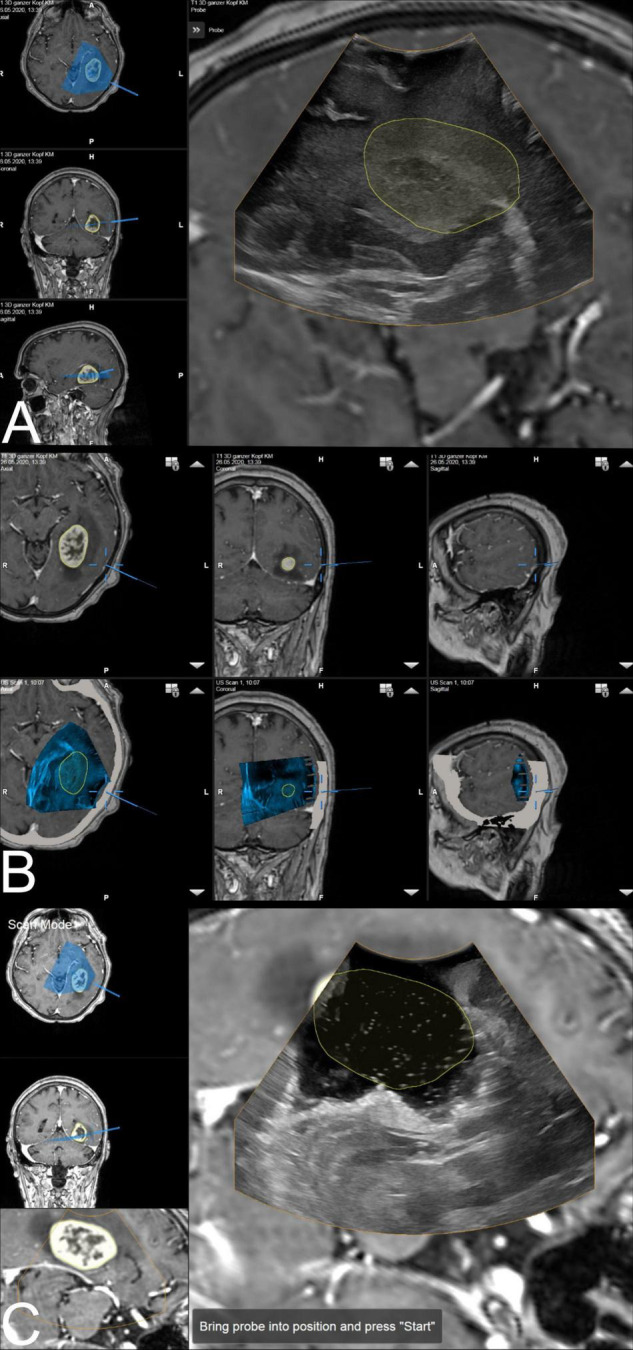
The ultrasound application: intraoperative display of i3D US over MRI data. (A) The first intraoperative ultrasound before dural opening is displayed as an overlay. The yellow line shows the tumor borders segmented on pre-MRI, which are not correspondent with i3D US, indicating brain deformation. Corresponding axial, coronal, and sagittal view of pre-MRI data is shown on the left side, including a visualization of localization and orientation of the recent i3D US. (B) After i3D US acquisition, the ultrasound application automatically displays pre-operative imaging (upper row: pre-MRI) and intraoperative imaging (lower row: i3D US as an overlay in pre-MRI) simultaneously in an axial, coronal, and sagittal slice depending on the position of the navigated transducer (cursor). Besides this, the arrows on the right side of each view allow for scrolling through the co-registered i3D US and pre-MRI sets to further explore the data. (C) The second navigated intraoperative ultrasound after resection (post-US) is displayed as an overlay on the pre-MRI. Analogous to (A), the tumor outlines based on the pre-MRI data are visualized in yellow demonstrating the tumor boundaries to be completely within the resection cavity. For comparison of live ultrasound images and pre-MRI data, the scan mode view is chosen. In this case, the left-hand side of the image shows the axial and coronal view, as well as the inline view of the pre-MRI dataset, on which the ultrasound data is superimposed, without the overlay.
